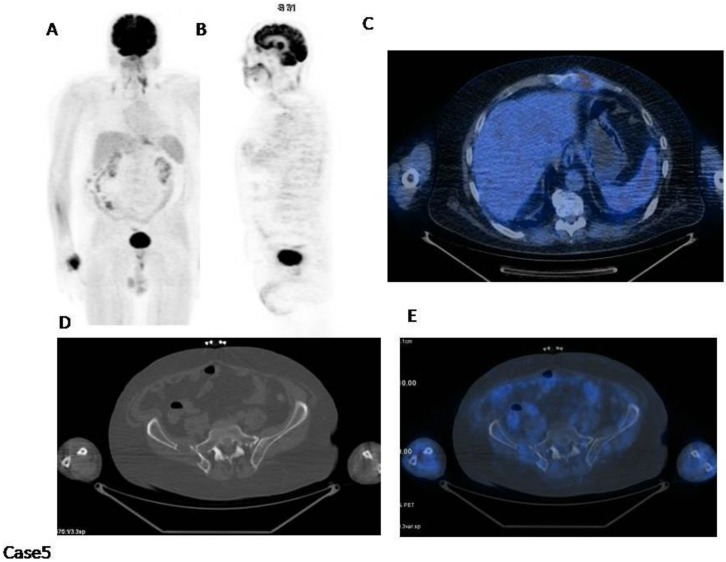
(A) MIP; (B) PET sagittal cut; (C) fused images axial cut; (D) CT axial cut; (E) fused images axial cut. There is a lytic lesion in the sternum involving surrounding tissues (C) and a cold lytic lesion in the right bony pelvis (E).
